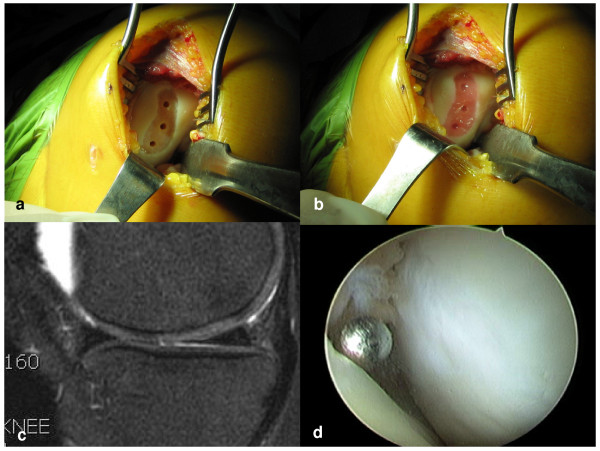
The gel-type ACI (Chondron™) surgery. a) Preparation of cartilage lesion, b) After injection of cell gel mixture, C) MRI one year postoperatively, D) Second-look arthroscopic view one year postoperatively.
